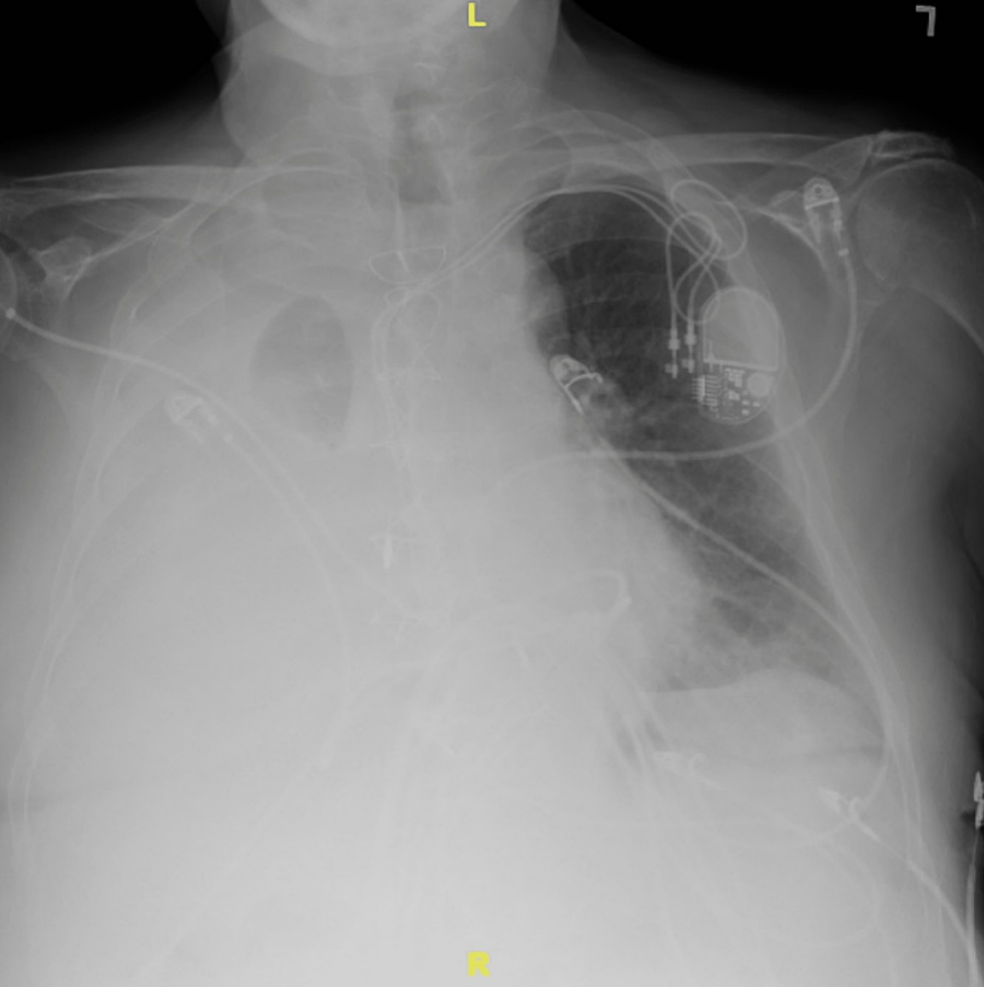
Chest radiograph with large right pleural effusion.
